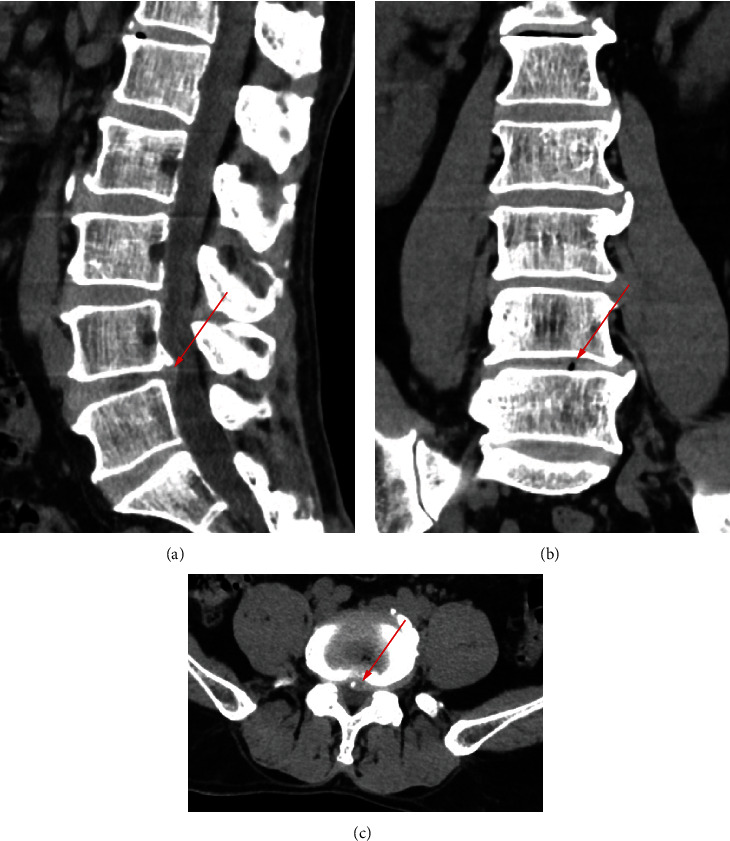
CT examination of a patient with nonspinal tuberculosis. The arrow points to the location of the lesion. (a) CT image in sagittal position. (b) CT image in coronal position. (c) CT image in cross-section.
